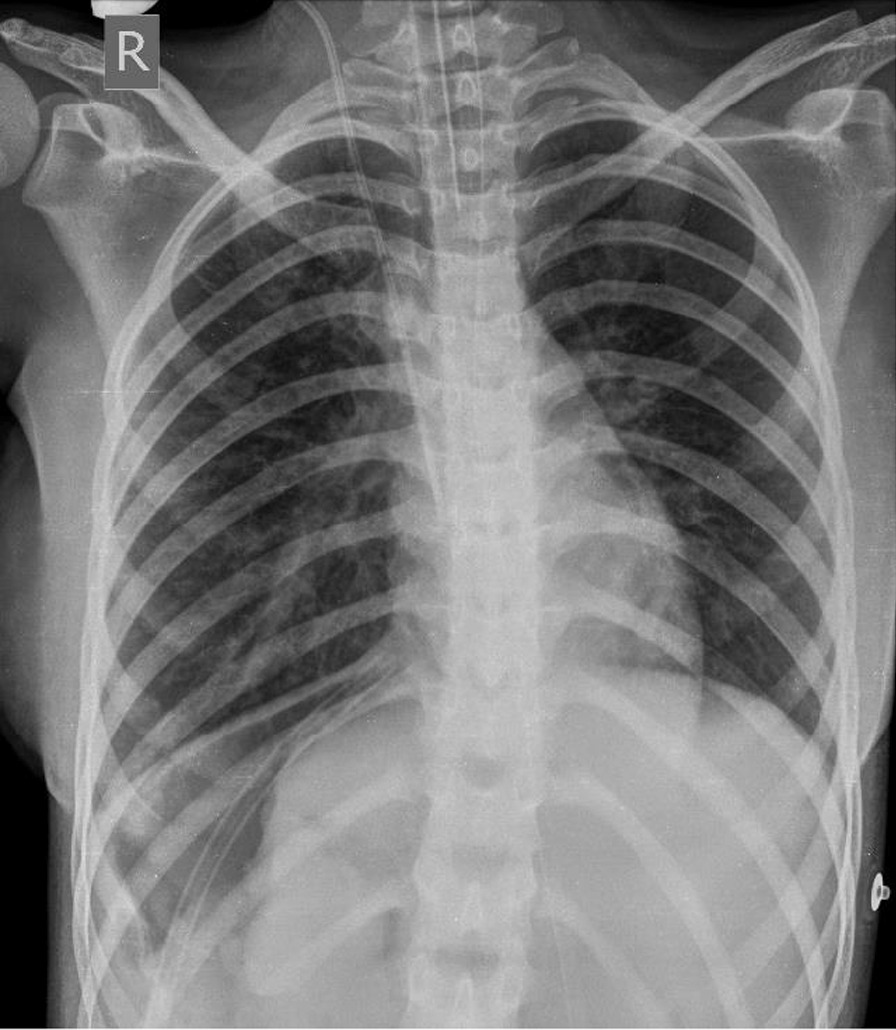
Chest X-ray after surgical resection of a huge undifferentiated embryonal sarcoma of the liver
